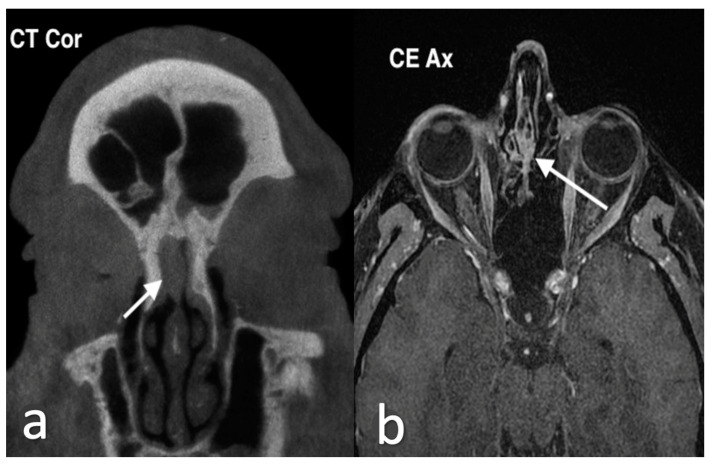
A nasal chondromesenchymal hamartoma in a patient with suspected rhinosinusitis. Coronal CBCT image (a) demonstrates, in the upper anterior third of the nasal septum, an oval lesion with well-defined margins and soft tissue density, extending in the upper third of both nasal cavities (arrow). No erosion of adjacent bony structure is visible. On MRI, in the T1 axial fat saturation image after intravenous Gd (b), a diffuse heterogeneous enhancement of the mass, with no enhancement of small cystic components, is appreciable (arrow).
